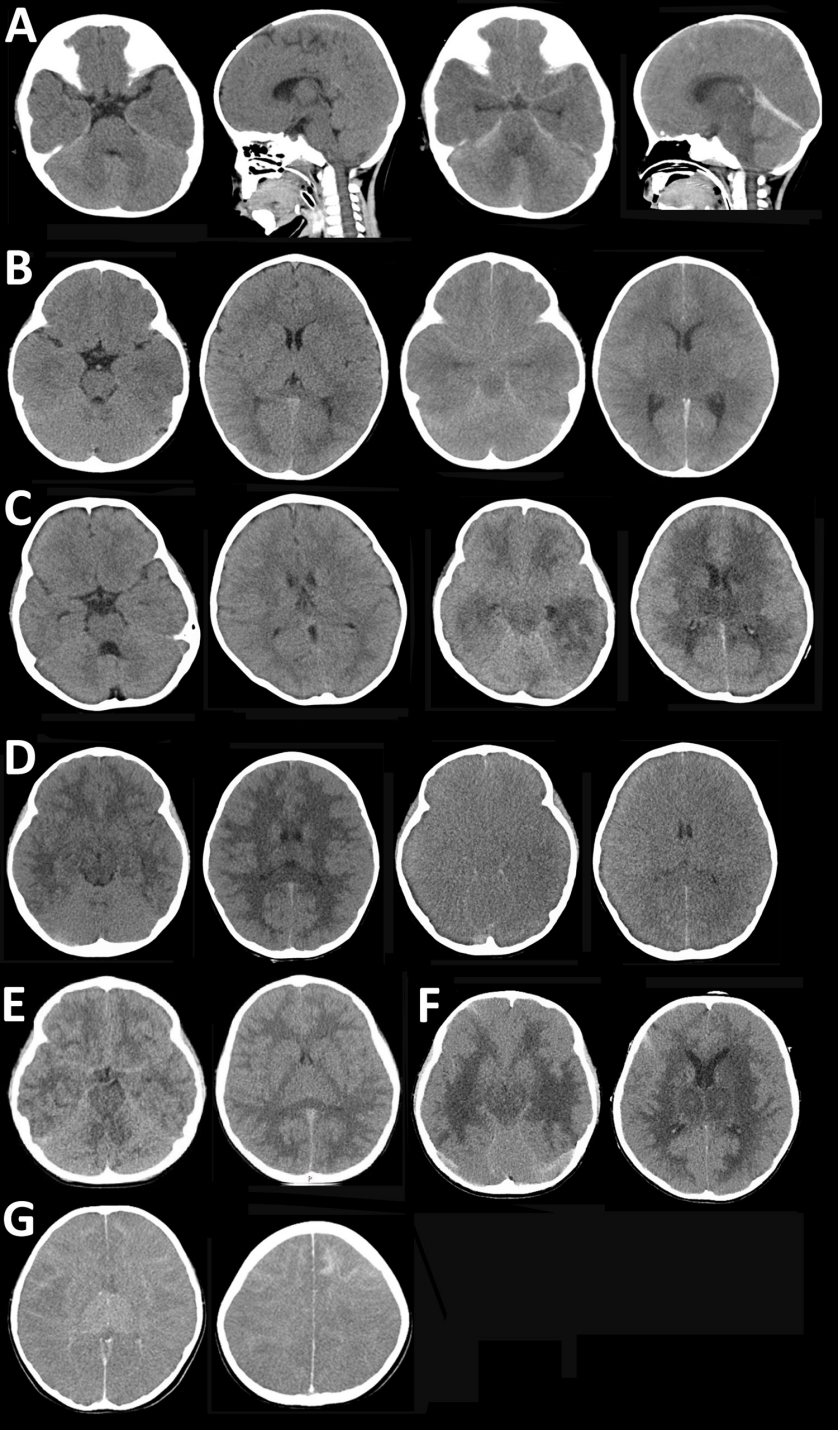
Brain computed tomography imaging for patients with SARS-CoV-2 acute shock with encephalopathy and multiorgan failure/acute fulminant cerebral edema (ASEM/AFCE) for study of life-threatening SARS-CoV-2–associated encephalopathy and multiorgan failure in children, Asia and Oceania, 2022–2024. A) Case-patient 1, showing diffuse cerebral edema (DCE) within 10 hours after onset of neurologic symptoms (left 2 images) and severe DCE and herniation at 18 hours after onset (right 2 images). B) Case-patient 6, showing mild cerebral edema within 4 hours after onset (left 2 images) and severe DCE at 23 hours after onset (right 2 images). C) Case-patient 15, showing DCE 3 hours after onset (left 2 images) and severe DCE with low-density involving the bilateral cerebral white matter and thalamus at 23 hours from the onset (right 2 images). D) Case-patient 19, showing DCE with low-density lesions in the bilateral cerebral white matter within 90 minutes after onset (left 2 images) and severe DCE at 10 hours after onset (right 2 images). E) Case-patient 18, showing mild cerebral edema on day 2 of illness; the patient had no further exacerbation of DCE. F) Case-patent 20, showing DCE with bilateral low-density lesions in the thalamus (left image) and periventricular cerebral white matter within 30 hours after onset (right image). G) Case-patient 23, showing DCE within 7 hours after onset (left image) and a high density of frontal subcortical white matter (right image).
